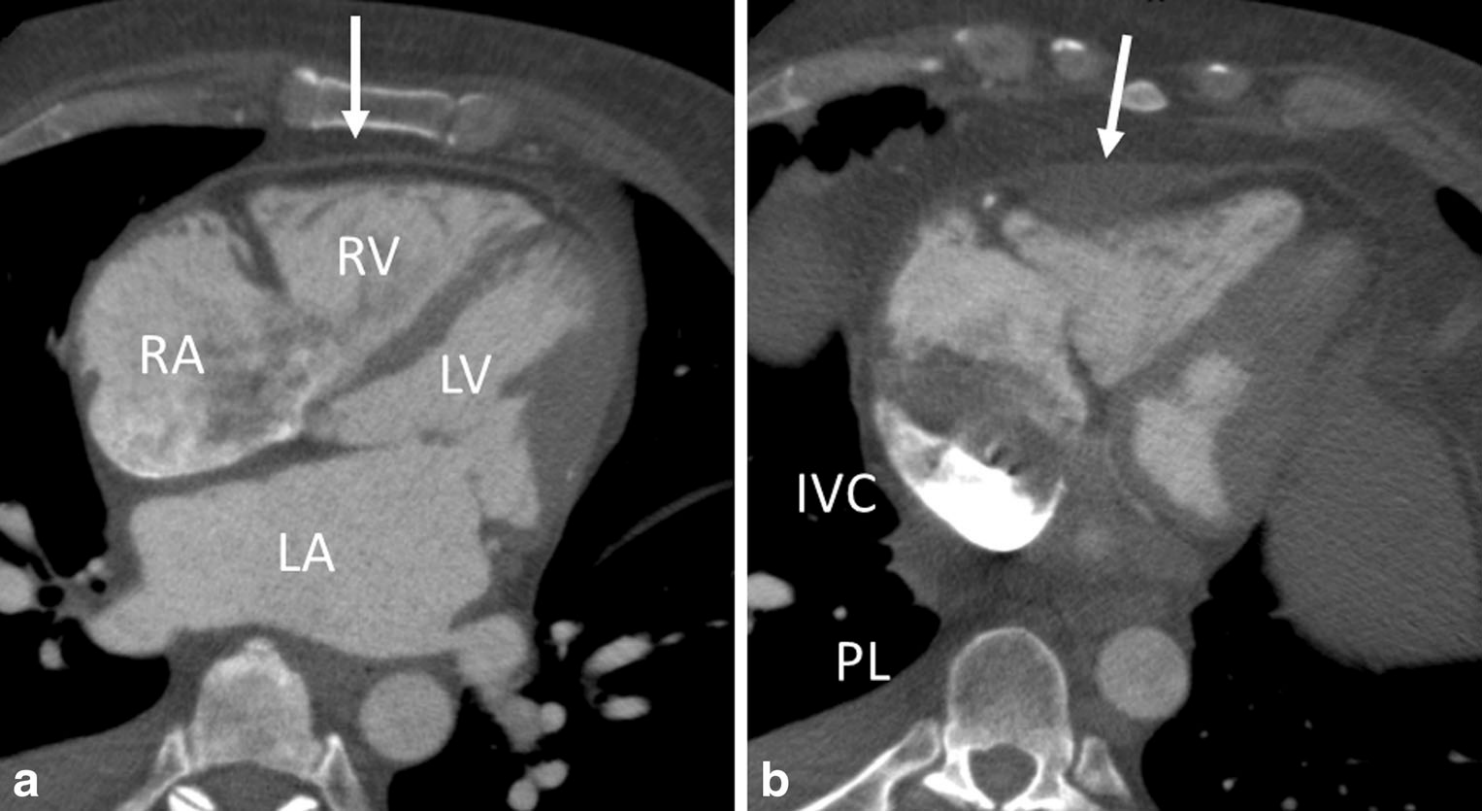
44-year-old male patient with constrictive pericarditis. (a-b) CT images in axial views. The pericardium is thickened (arrows). (a) The right atrium (RA) and left atrium (LA) are enlarged. (b) Note the abnormal shape of the right ventricle (RV) and left ventricle (LV), and inferior vena cava enlargement (IVC). Patient had pleural effusion (PL) as a sign of decompensation cordis. These are among signs of constriction
